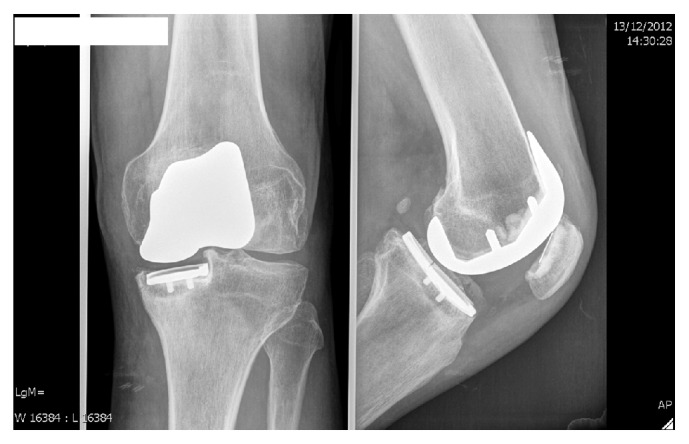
Anteroposterior and profile view X-rays of the knee 4 years after surgery. Arrow indicates fracture of the baseplate.
